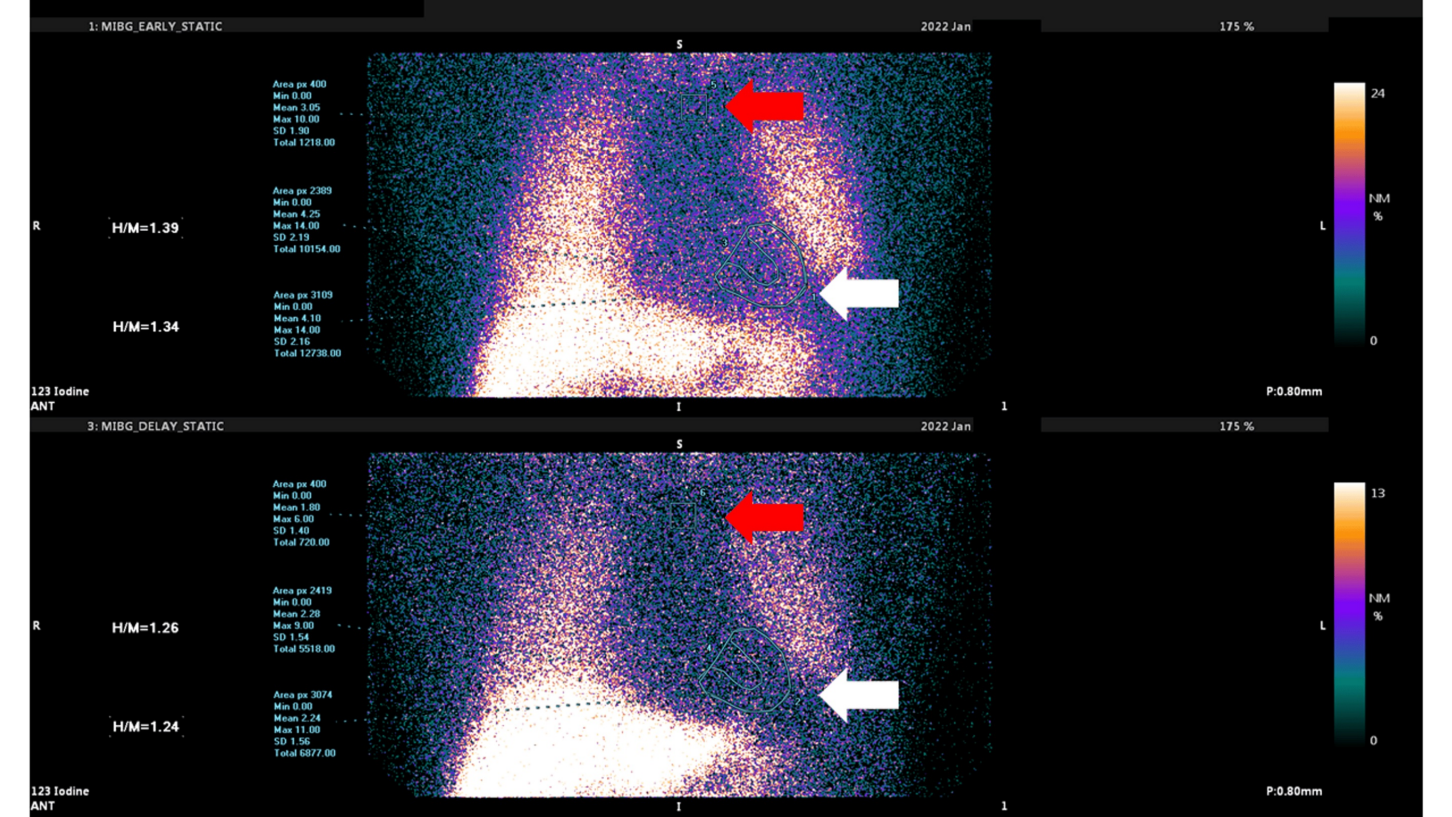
The early H/M ratio using 123I-MIBG myocardial scintigraphy. The heart (white arrows)/mediastinum (red arrows) (H/M) ratio was decreased in both the early (upper) and late (lower) images. H/M = heart-to-mediastinum; 123I-MIBG = 123I-meta-iodobenzylguanidine
